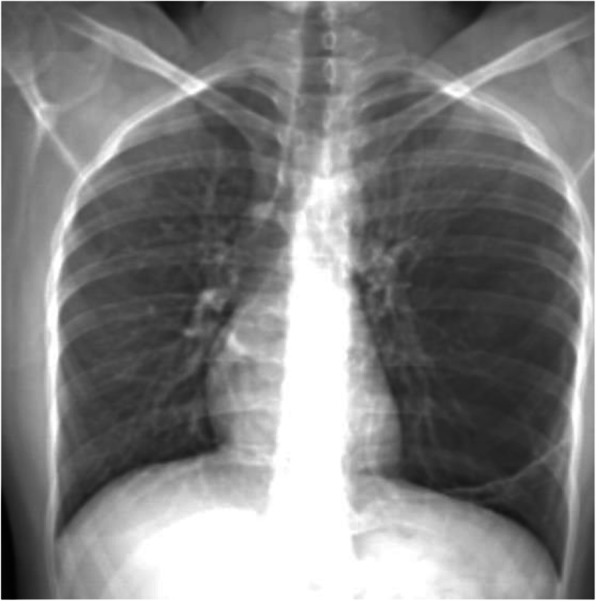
CT scout radiograph showed increased radiolucency involving mid and lower zones of the left lung with displacement of broncho-vascular markings with mild mediastinal to right side
